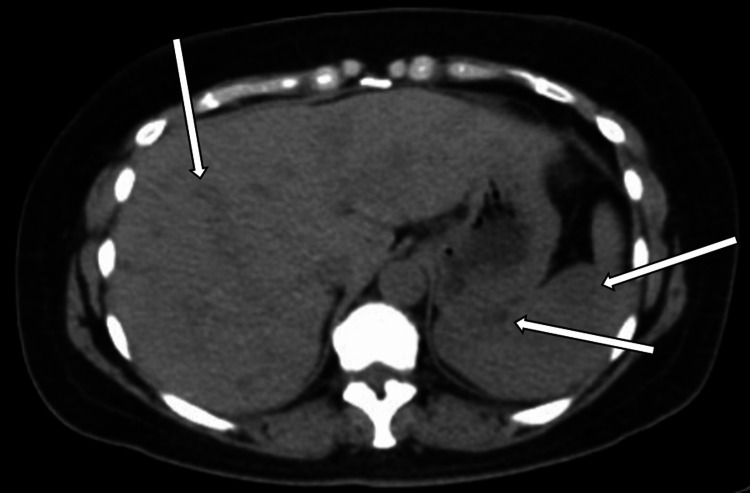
Abdominal CT scan showing multiple nodules of the liver and spleen (white arrows) CT: computed tomography
